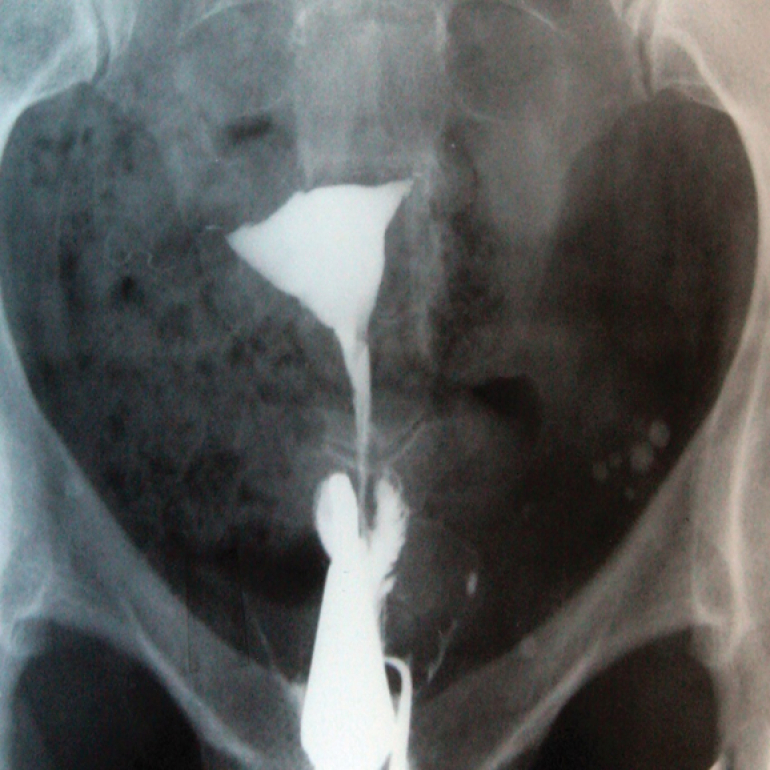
Normal uterine cavity. The concentration of tiny phleboliths on the left side of the pelvis and outside of the uterine cavity. Phleboliths are frequently seen in pelvic x-ray and have no clinical value.
